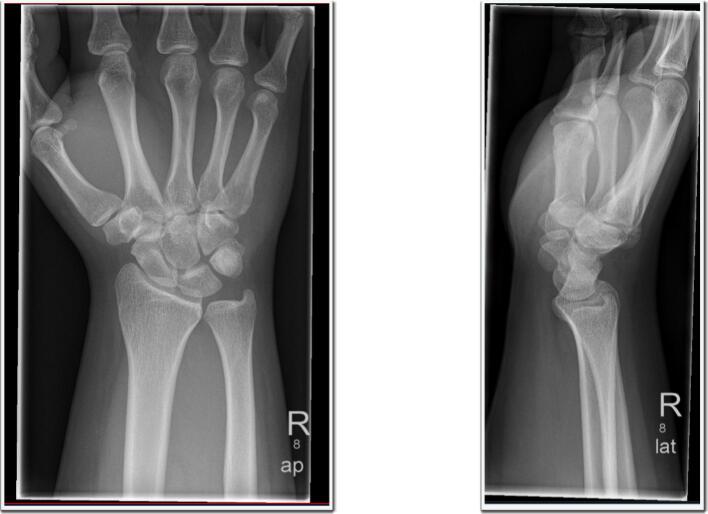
Radiographic evidence of spontaneous pisiform repositioning in two planes (BG-Klinik, Halle, Saale).
